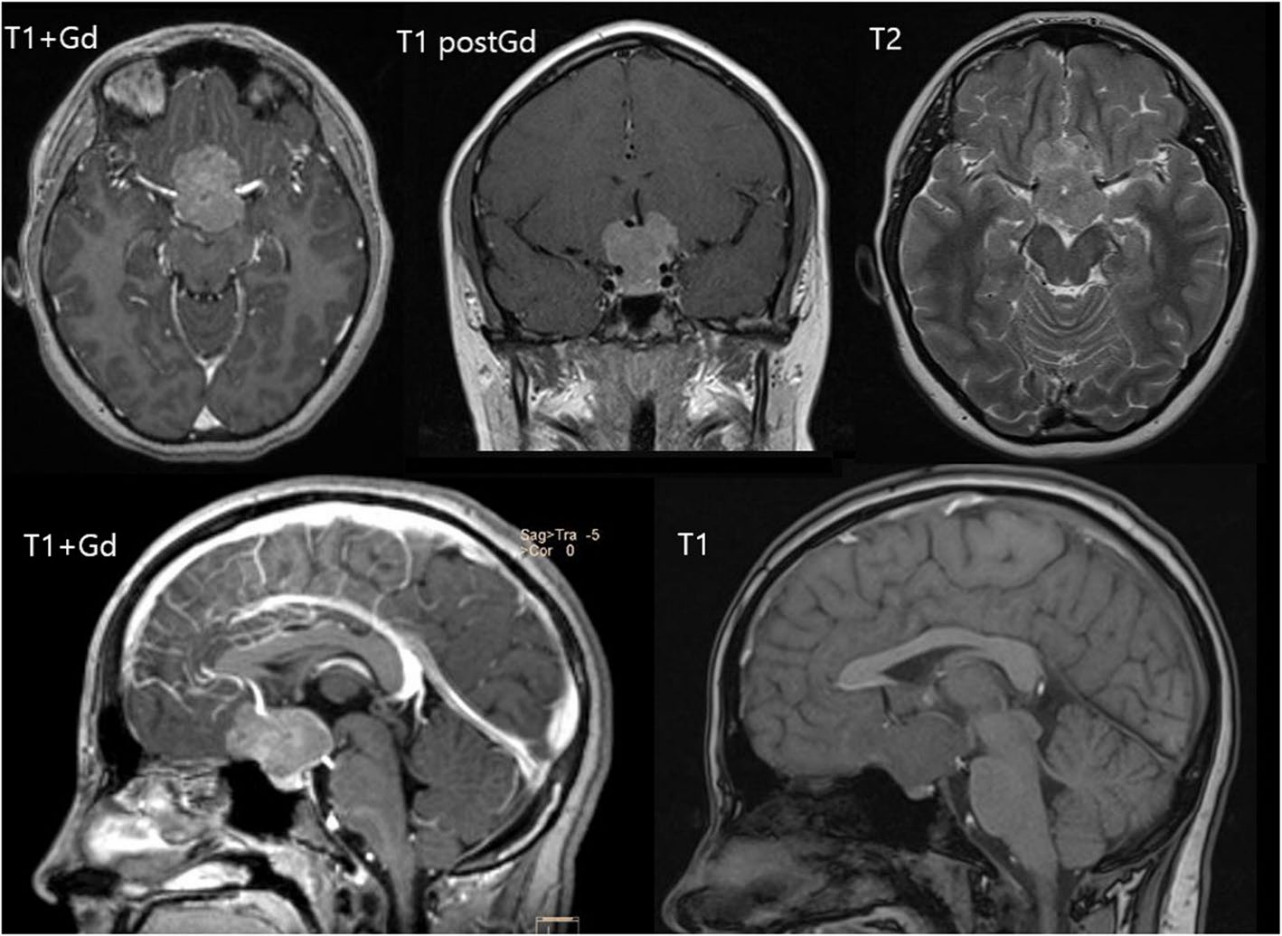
Initial MRI. MRI shows suprasellar tumor that dislocates undamaged 3rd ventricle floor, optic chiasm, and optic nerves rostrally and pituitary gland with its stalk backward. The tumor seems to have no dural attachment or signs of brain invasion
